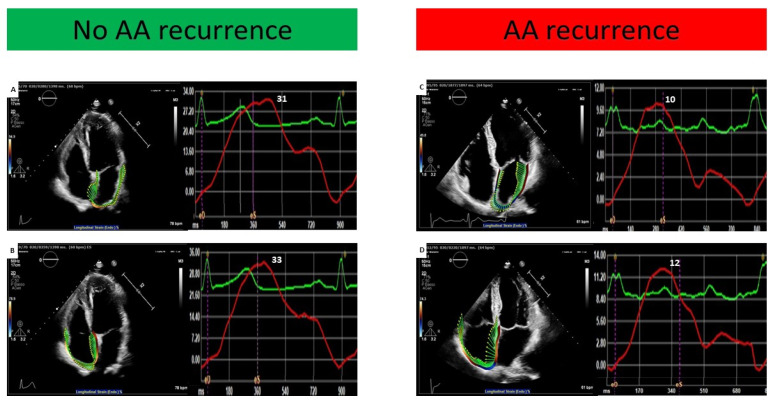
Echocardiographic images with atrial strain analysis performed in two patients included in our study. On the left, the pre-AFL ablation atrial strain analysis of a patient who did not experience AAs at follow-up shows normal values of LASr (A) and RASr (B). On the right, pre-AFL ablation atrial strain analysis of a patient with documented AA recurrence at follow-up shows low LASr (C) and RASr (D) values. Abbreviations: AAs, atrial arrhythmias; AFL, atrial flutter; LASr, left atrial strain reservoir; RASr, right atrial strain.
